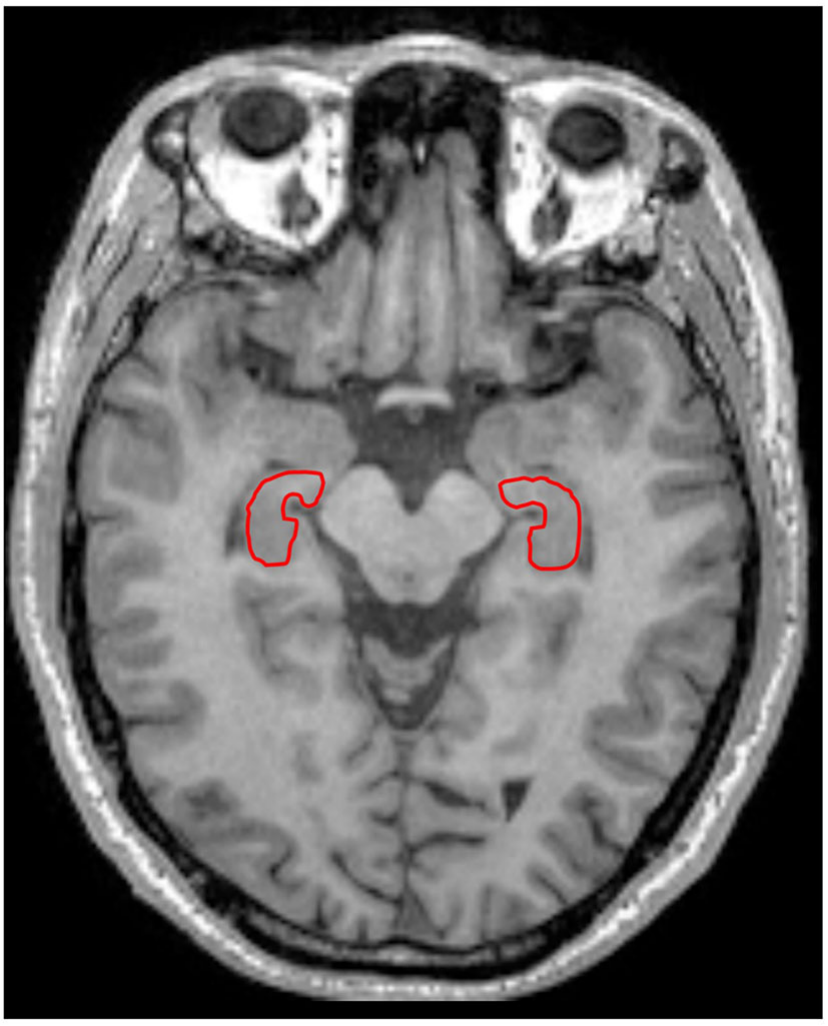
A 67-year-old male healthy control. The left and right hippocampus are outlined in red line in T1-weighted imaging.
